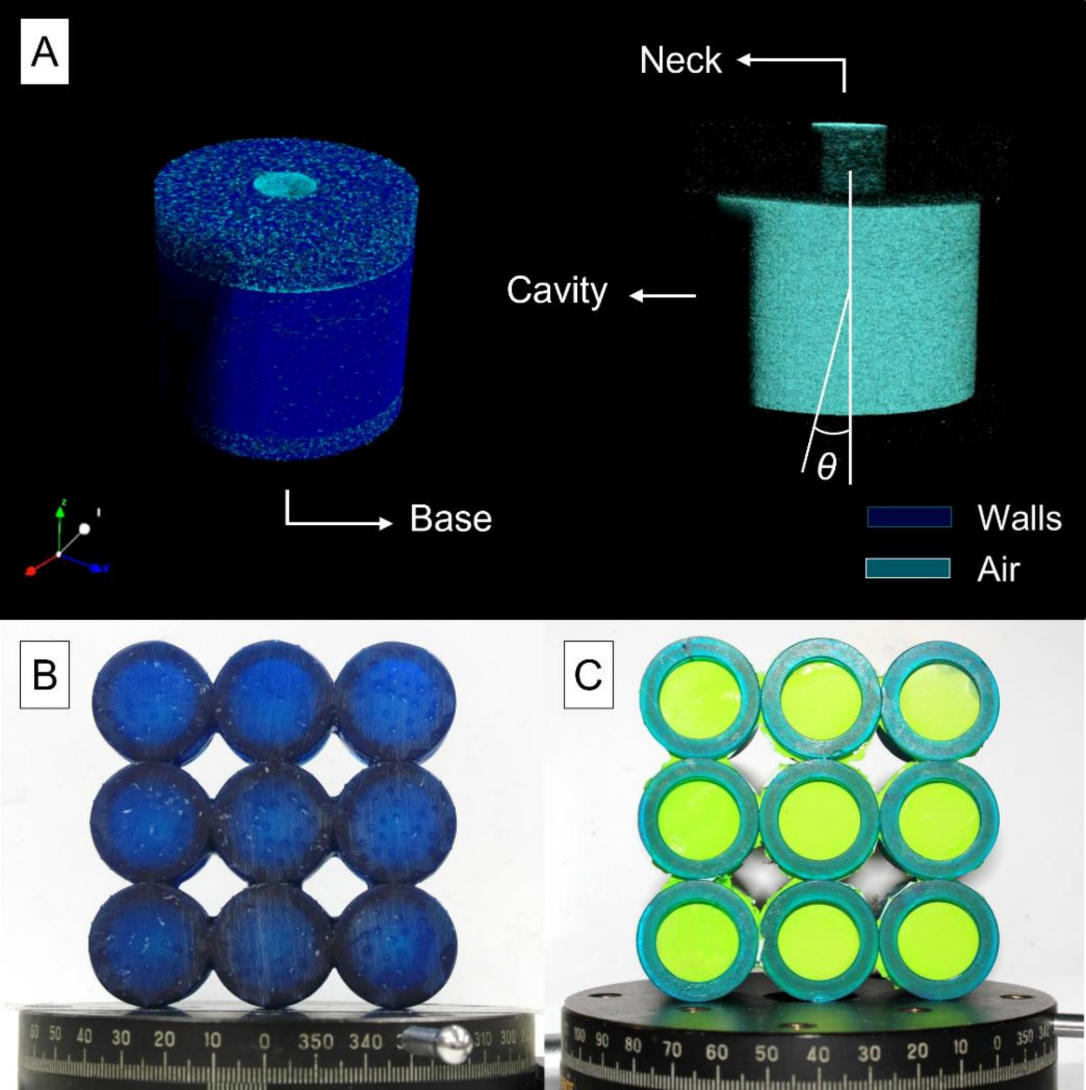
(A) Representation of the geometry of a Helmholtz resonator (HR), indicating its multiple components (neck, cavity, and base – explicitly mentioned because this is replaced by a membrane), and indicating in different shades of blue the walls and the air inside the resonator. The definition of the angle of incidence (*θ*) of the acoustic field is shown in the right-hand-side panel of (A), leading to *θ* = 0° when the pressure field impinges directly onto the base (or membrane) of the HR. Representations obtained via X-ray micro-computed-tomography (details in Supplementary Material). (B) and (C) show, respectively, the 3D-printed HR and membrane-coupled HR acoustic metamaterials from the front view (*θ* = 0°). (C) Also shows the 3D-printed rings that sit on top of the membrane. Scale bars, 10 mm.
